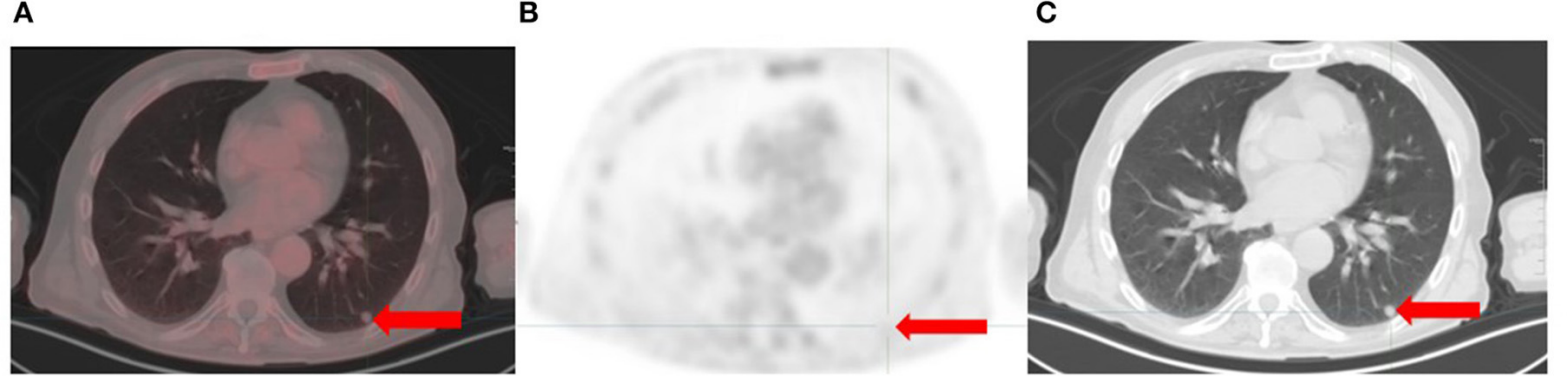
Recurrent melanoma with a 9 mm left lung nodule (arrows). There is no metabolism on the [18F]FDG PET/CT trans-axial fused (A) and PET only (B) images. CT features are suspicious for malignancy (C).
